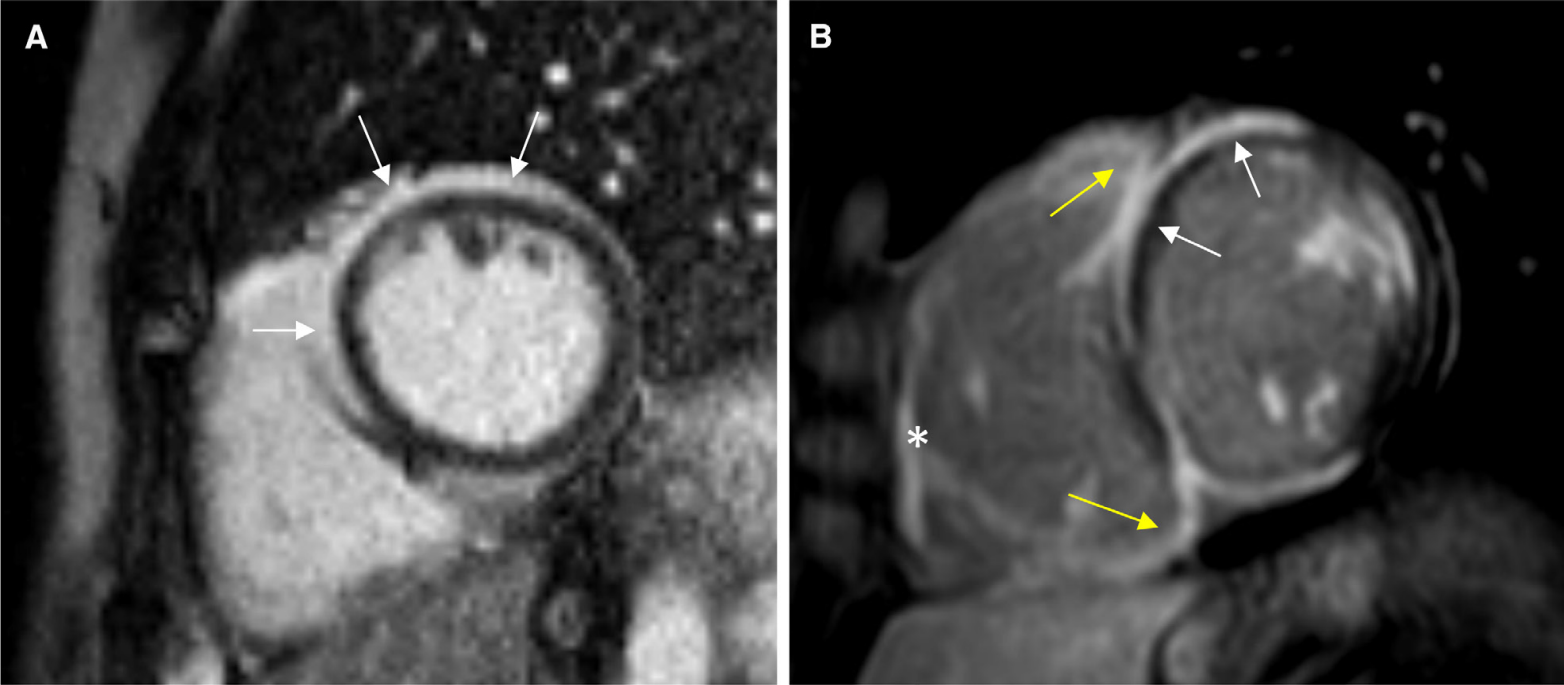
Contrast-enhanced magnetic resonance imaging phenotypes of cardiac sarcoidosis featuring the distribution of late gadolinium enhancement (LGE). The images present short-axis views of both ventricles. A, Demonstrates ring-like LGE^17,18^ with continuous subepicardial enhancement in the basal anterior and anteroseptal left ventricular segments (arrows), extending into parts of the inferoseptal and anterolateral segments. B, Shows right ventricular free-wall LGE (asterisk), the hook-sign LGE at septal insertions (yellow arrows), as well as septal and subepicardial LGE (white arrows), demonstrating multifocal myocardial involvement and thus all 4 features of pathology-frequent LGE.^20.^
